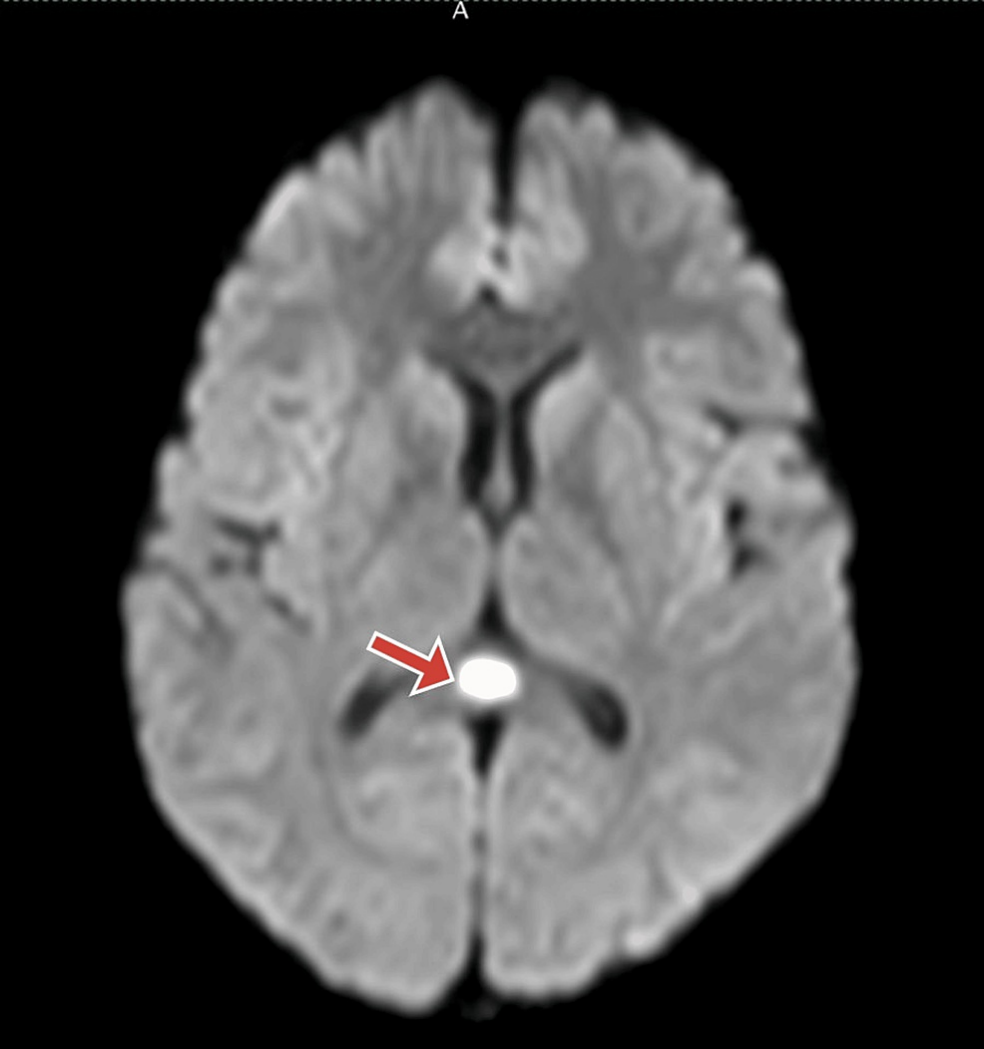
MRI of the brain The axial diffusion-weighted image shows a small central lesion involving the splenium of the corpus callosum with increased signal intensity.
